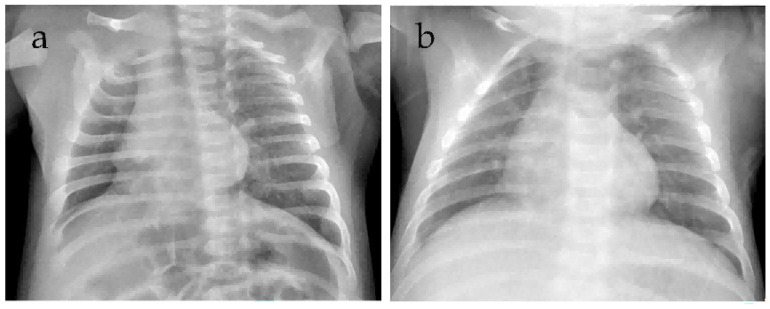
Chest radiograph images of Case 2 (a,b). Image (a) obtained at admission, demonstrating patchy opacity. Image (b) obtained 2 months after discharge, showing clear lung fields.
